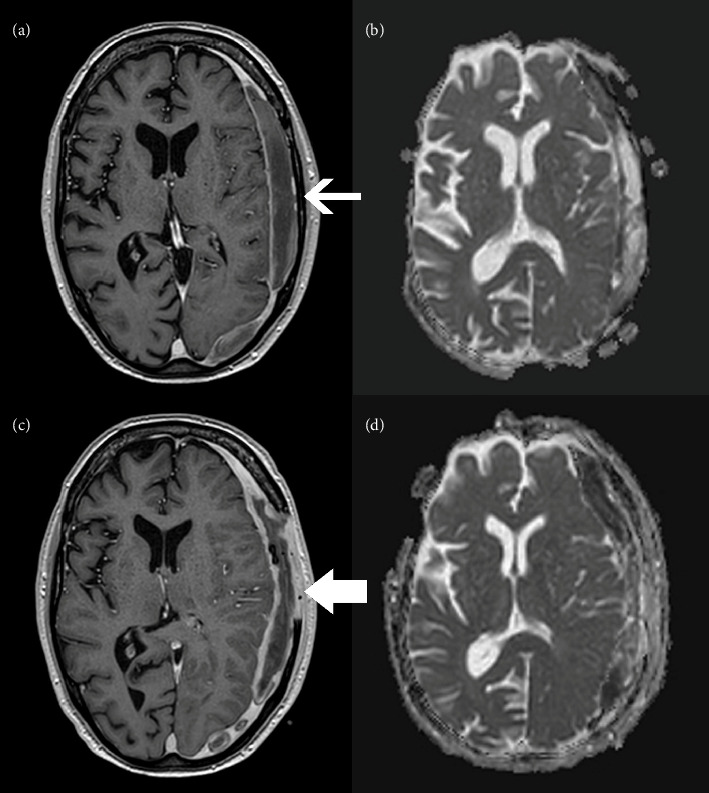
(a) Image from axial view MRI demonstrating mild rim enhancement (narrow arrow) and (b) diffusion restriction after burr hole surgery. (c) Repeat MRI images of similar axial views now demonstrating more marked rim enhancement (wide arrow) and (d) diffusion restriction following craniectomy. These findings are consistent with subdural empyema.
